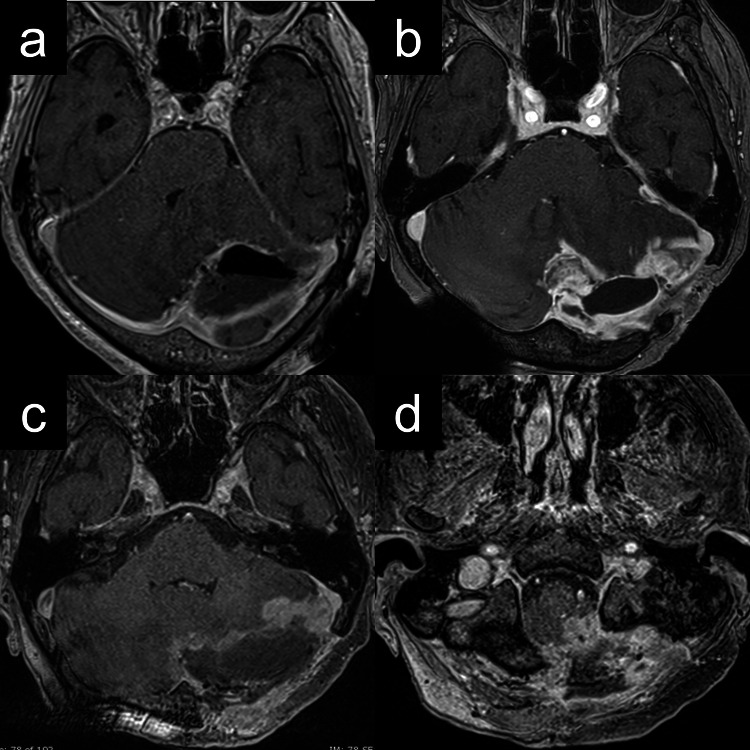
Clinical course of tentorial meningioma after initial resection on gadolinium-enhanced T1-Weighted magnetic resonance imaging (MRI). Tumor resection with suboccipital craniotomy was performed, achieving Simpson grade II resection (a). Three months later, MRI revealed massive tumor recurrence within the resection cavity (b). Although a second resection was performed, tumor progressed in the resection cavity and around the foramen magnum, suggestive of dissemination (c, d)
